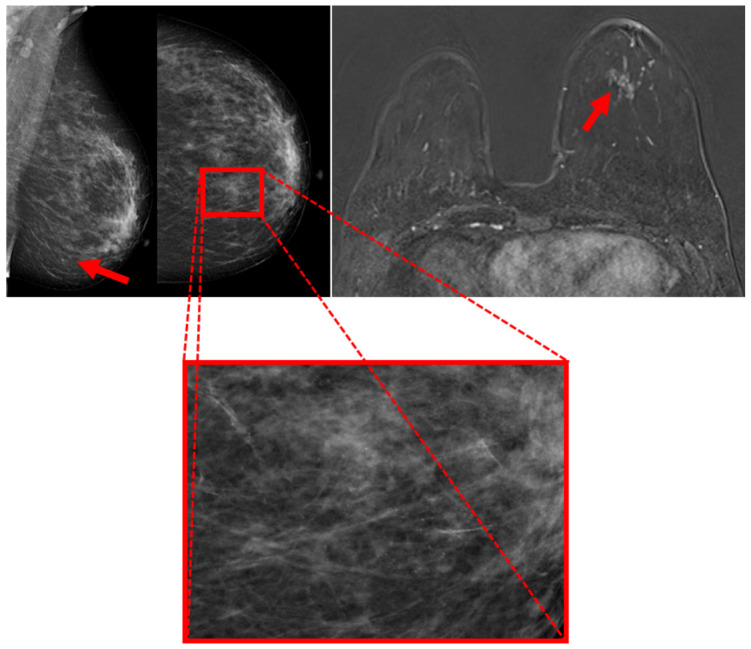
MLO mammogram, CC mammogram, and DCE-MRI images. Red arrows indicate the suspicious microcalcification area on mammographic images, and the red square indicates the corresponding contrast-enhancing area on DCE-MRI. MLO: mediolateral oblique; CC: craniocaudal; DCE-MRI: dynamic contrast-enhanced magnetic resonance imaging.
